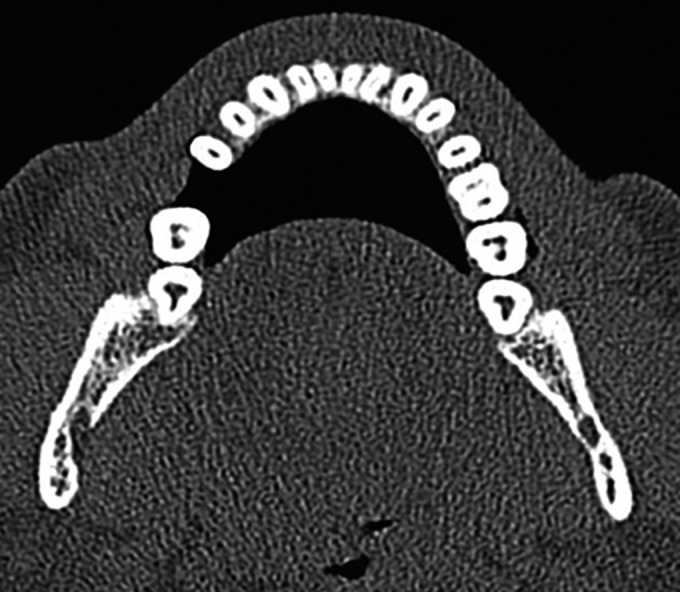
CBCT image of C-shaped canal system in both mandibular left and right molar teeth
